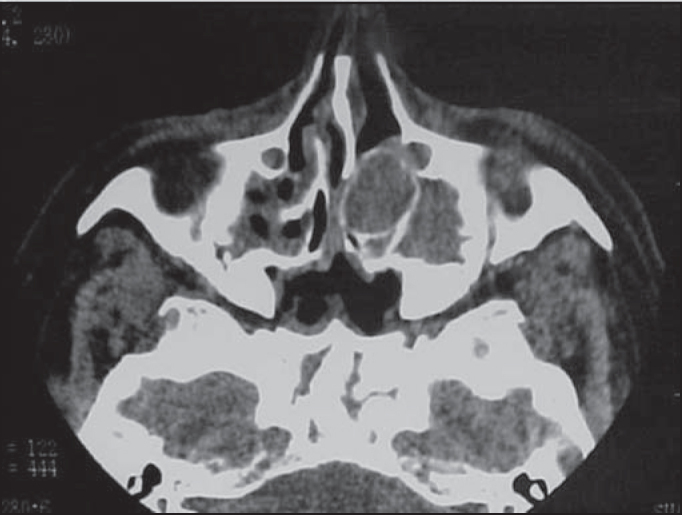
Paranasal sinuses CT scan (axial section) showing pansinusitis and mucopyocele of left middle concha.
